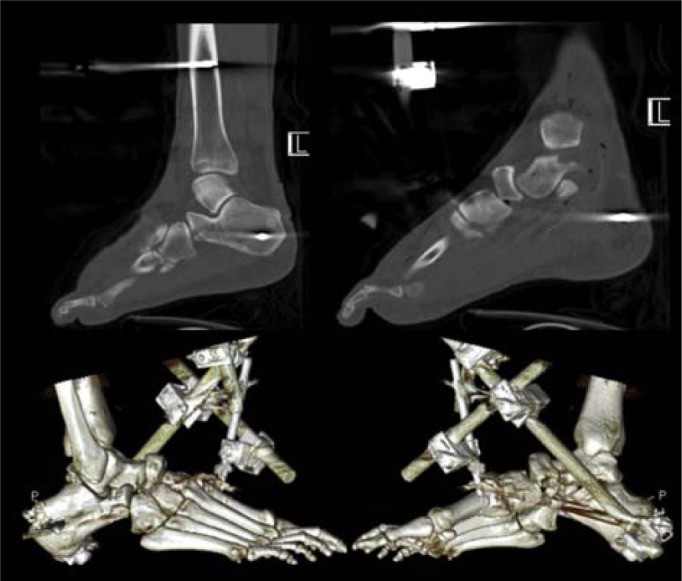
Computed tomography scan showing the unstable calcaneocuboid and naviculocuneiform joints, with a partially extruded navicular bone. Fractures of the cuboid and anterior calcaneal process were also defined.
